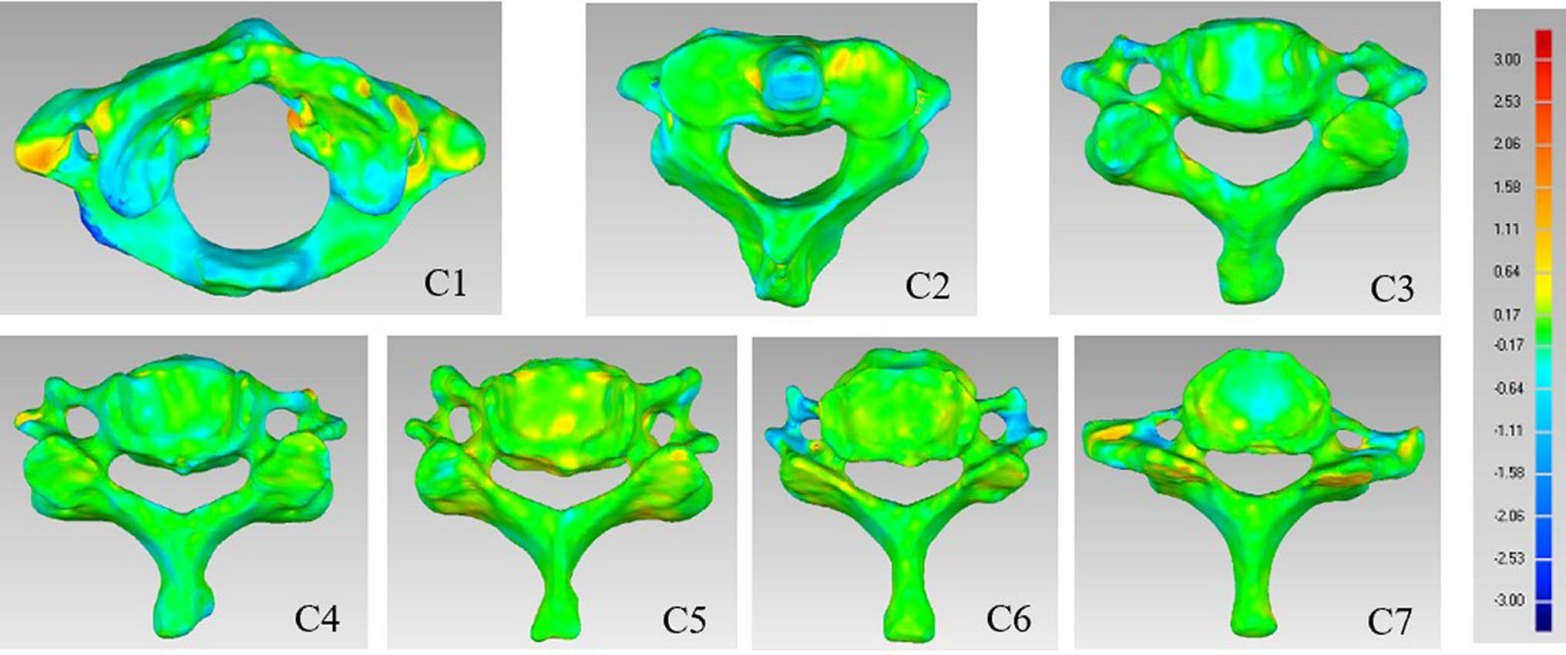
Superimposition of CBCT models with corresponding CT models of each cervical vertebra in the neutral position. Deviations between the CBCT and CT models are indicated with the color bar. Units, mm.
